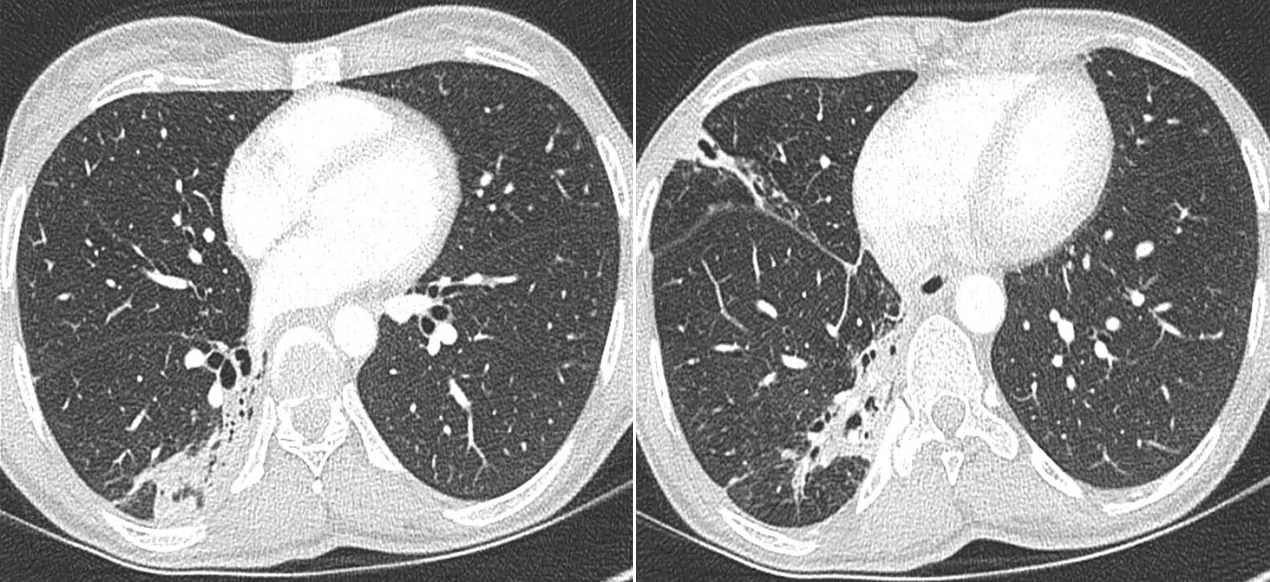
Computed tomography of the chest demonstrating post-treatment structural lung changes following multidrug-resistant tuberculosis. Patchy areas of consolidation, atelectasis, and bronchiectasis predominantly involving the right middle and lower lobes are consistent with sequelae of prior tuberculous disease.
